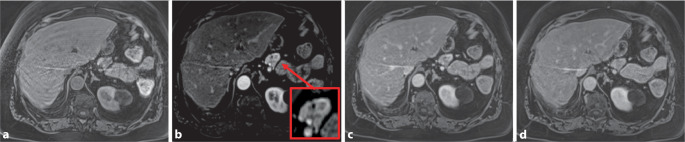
#### PET-CT mittels ^68^Ga-DOTATOC
